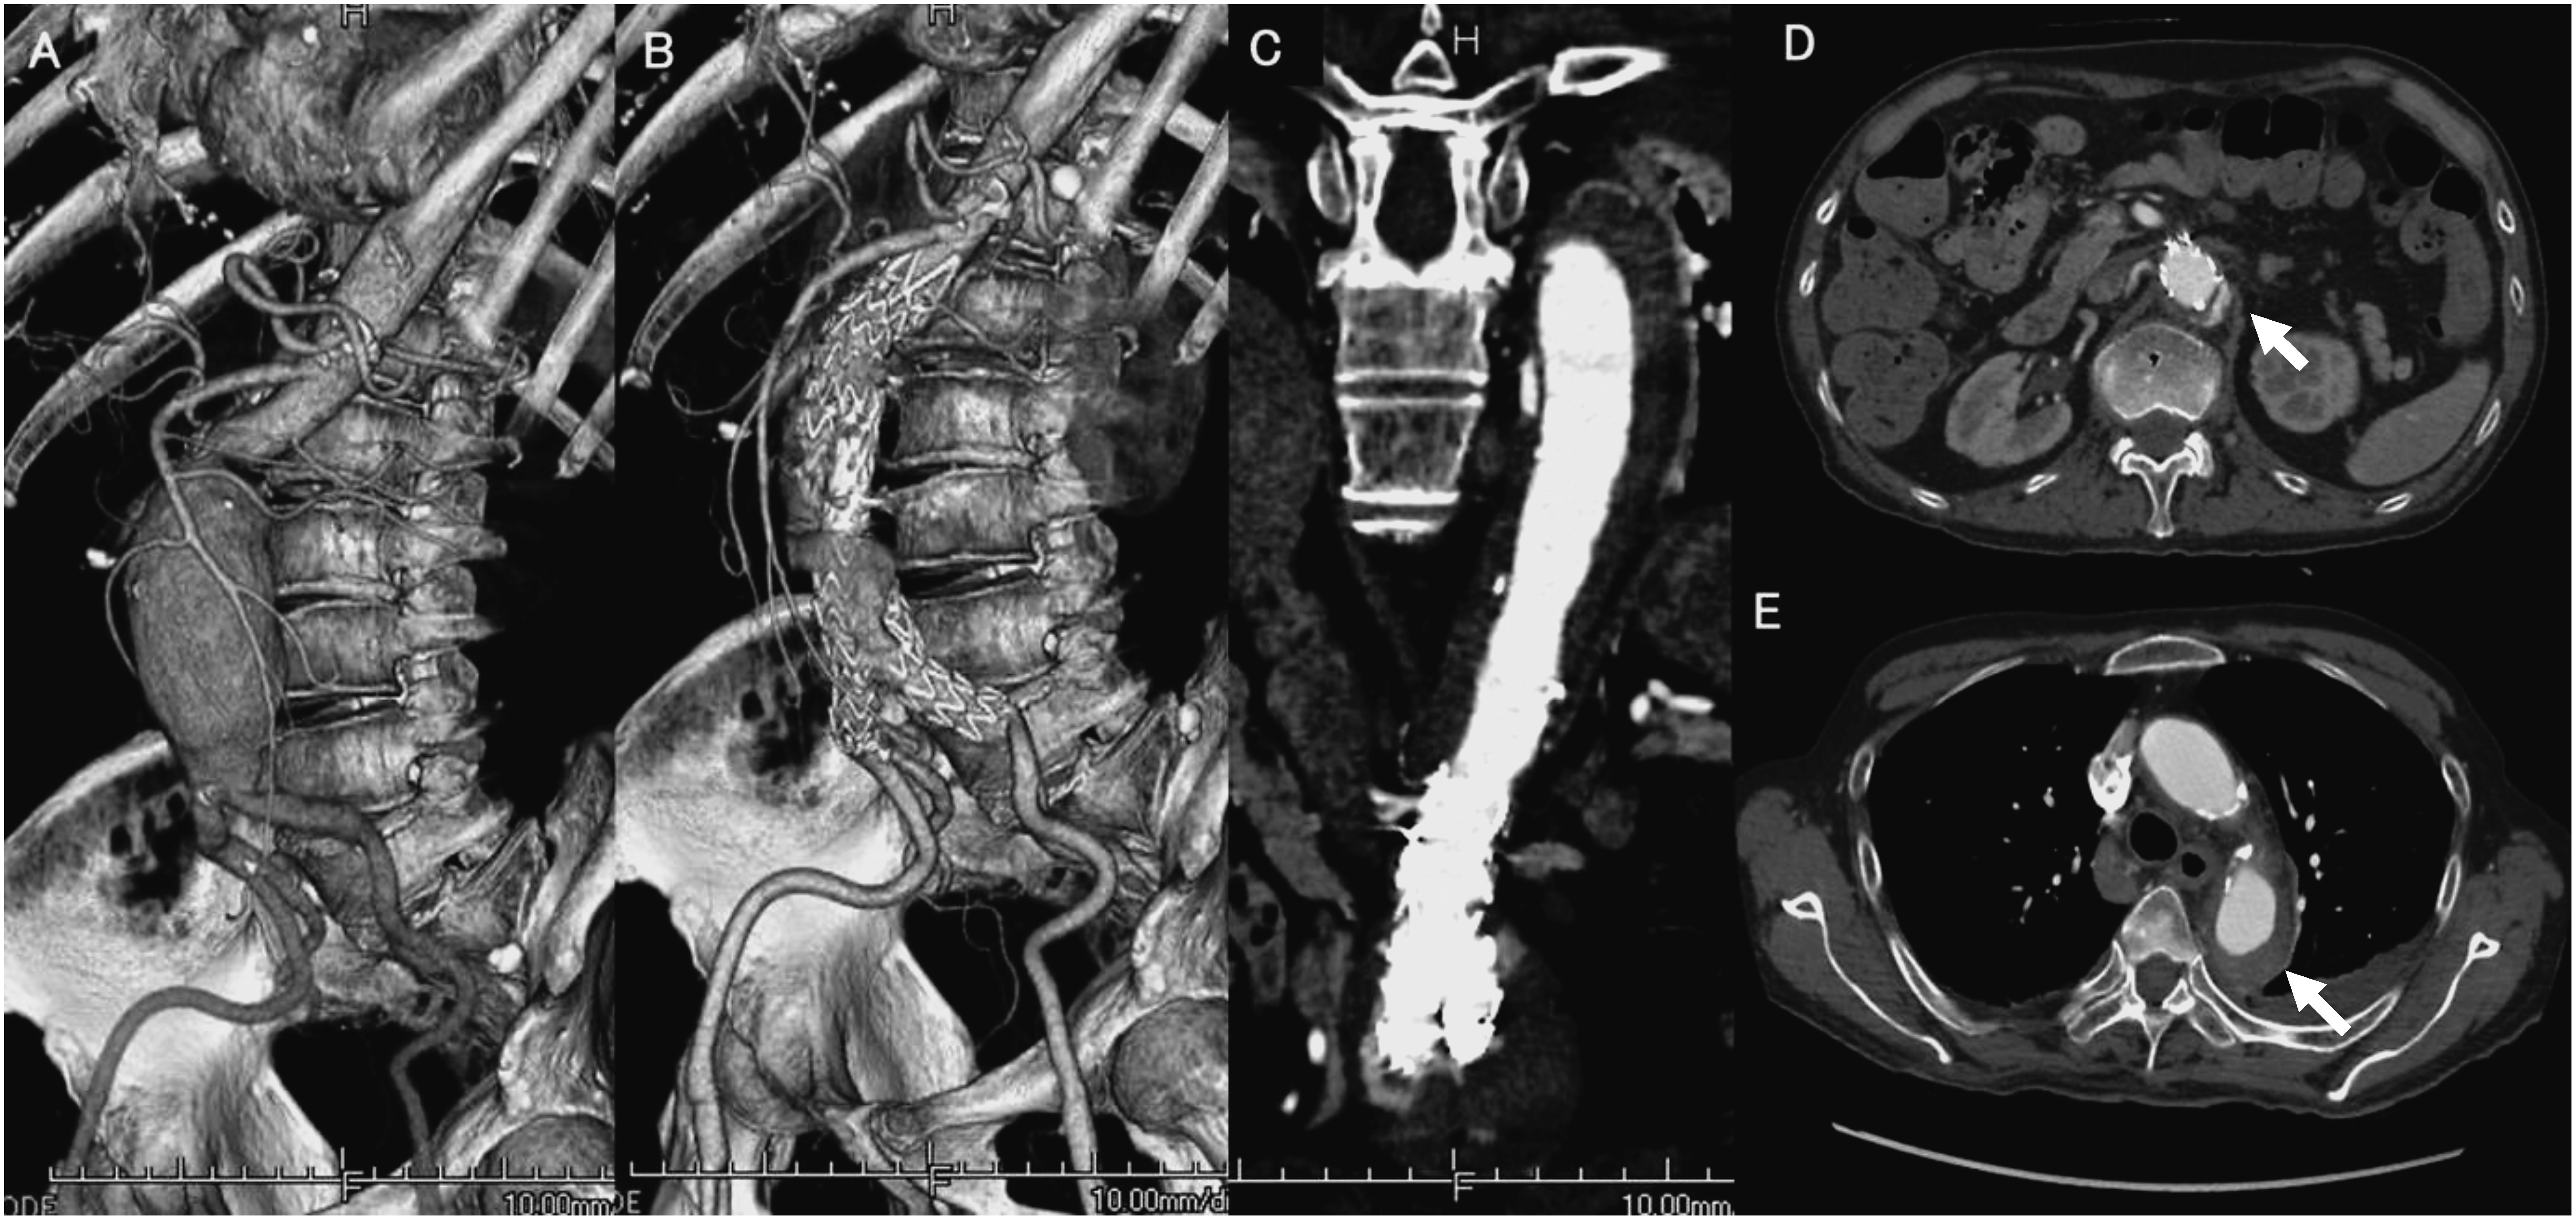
Fig. 2 (A) Preoperative three-dimensional computed tomography angiogram (3DCTA) revealing no calcification or atherosclerosis in the proximal neck. (B–E) Postoperative CTA. (B) 3DCTA showing the stent graft with proximal bare stents. (C) Multiplanar reconstruction revealing type B aortic dissection. (D) The axial plane at the proximal edge level of the stent graft. The white arrow shows the dissection entry. (E) The axial plane at the level of the left subclavian artery. The white arrow shows the thrombosed false lumen.
